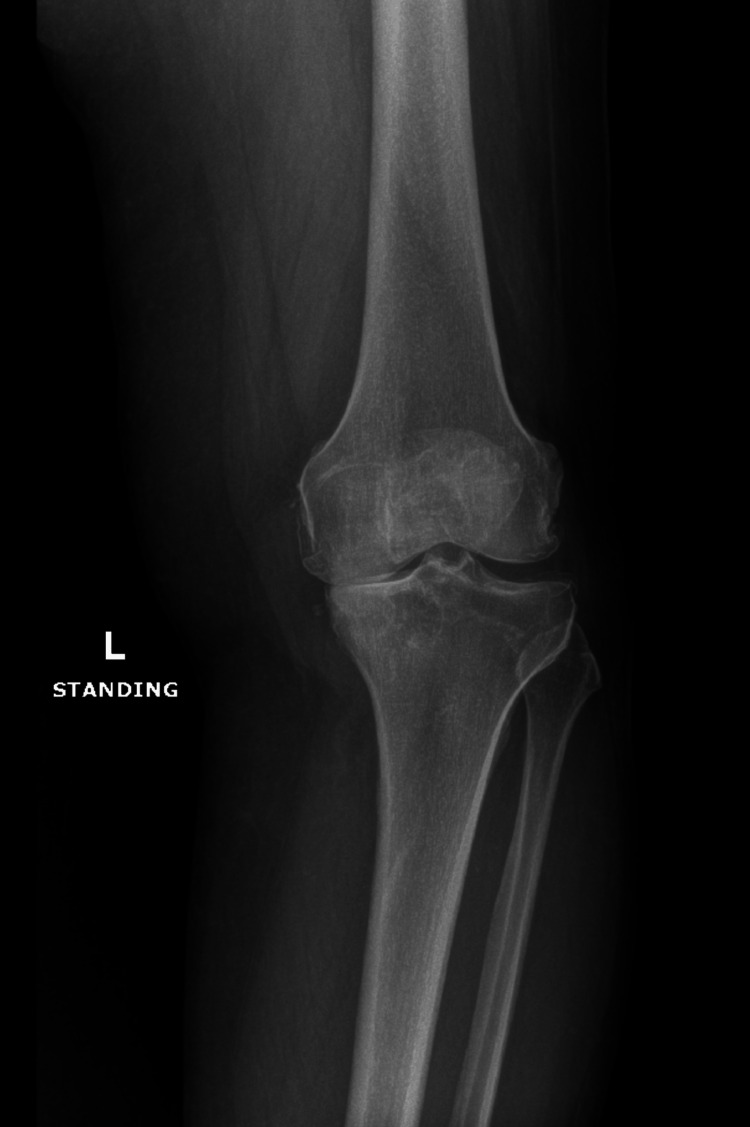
X-ray showing anterior posterior view of the patient's left knee.
